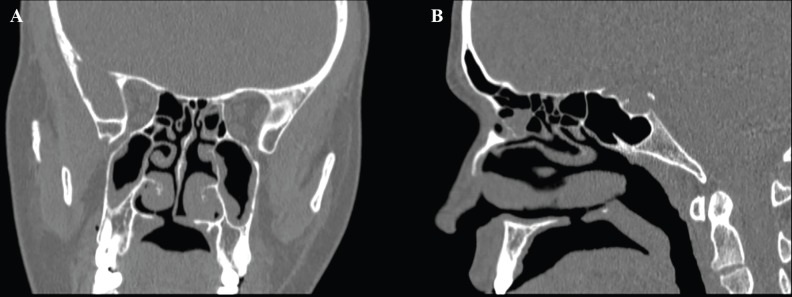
Palatal CT scan showing palatal fistula in an otherwise normal palate of the patient; Palatal arch is normal; coronal view (a), axial view (b)
